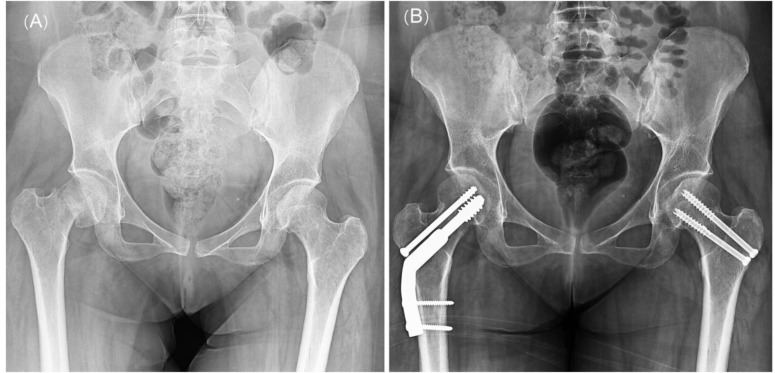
(A) Pre-operative radiograph of the femur showing right femoral neck fracture. Left femoral neck fracture line was only identifiable in the MRI; (B) Post-operative radiograph of the femur, showing the management of right femoral neck fracture with CRIF and DHS and the management of left femoral neck fracture with CRIF and screw.
